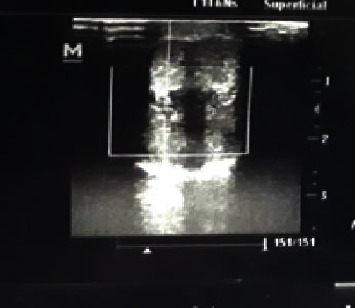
Demonstrating the Doppler ultrasonography with no flow to cavernous arterial flow.
